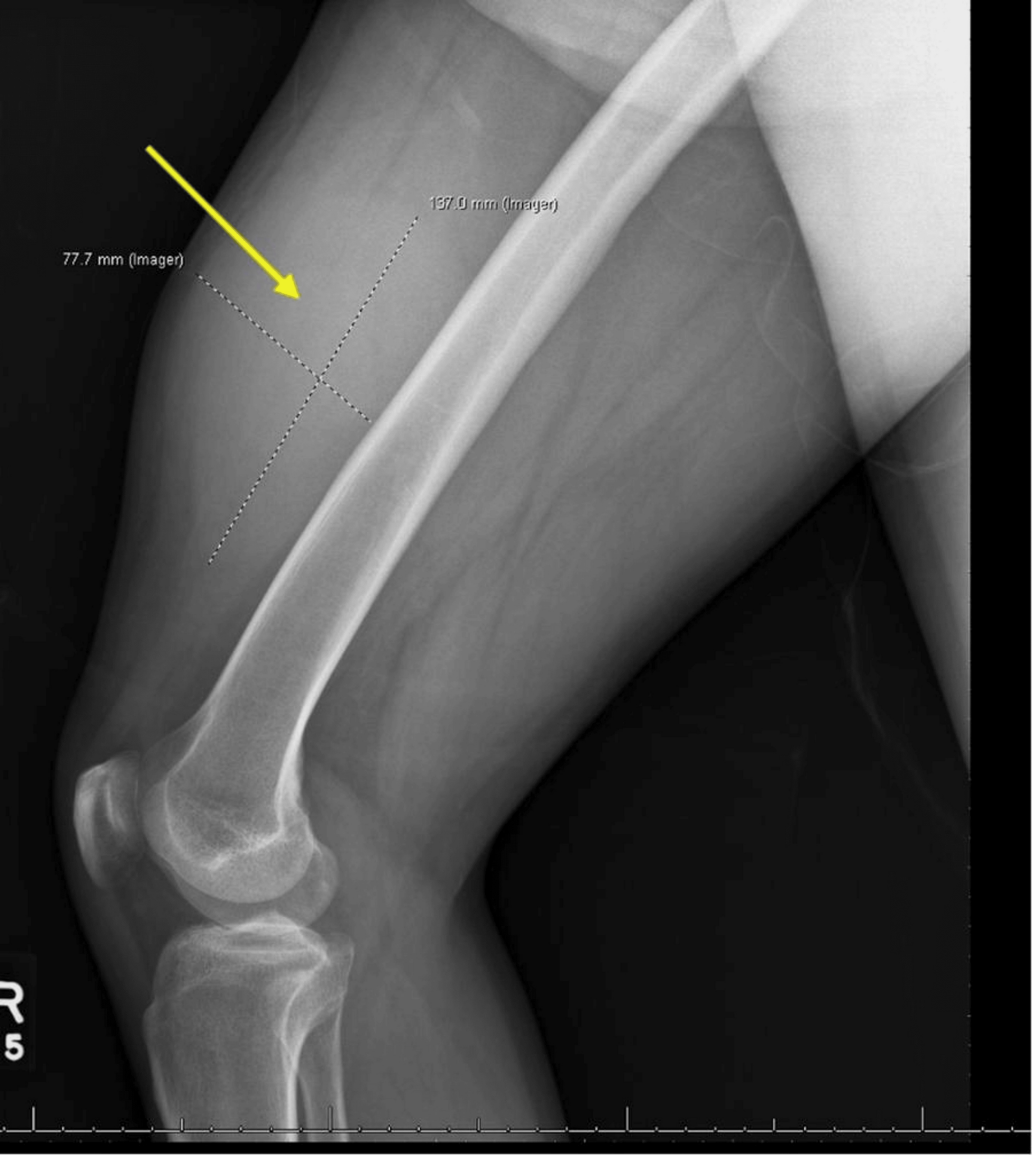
X-ray of the right femur revealed a 77.7 x 137 mm mass (arrow) and ruled out a femoral fracture.
